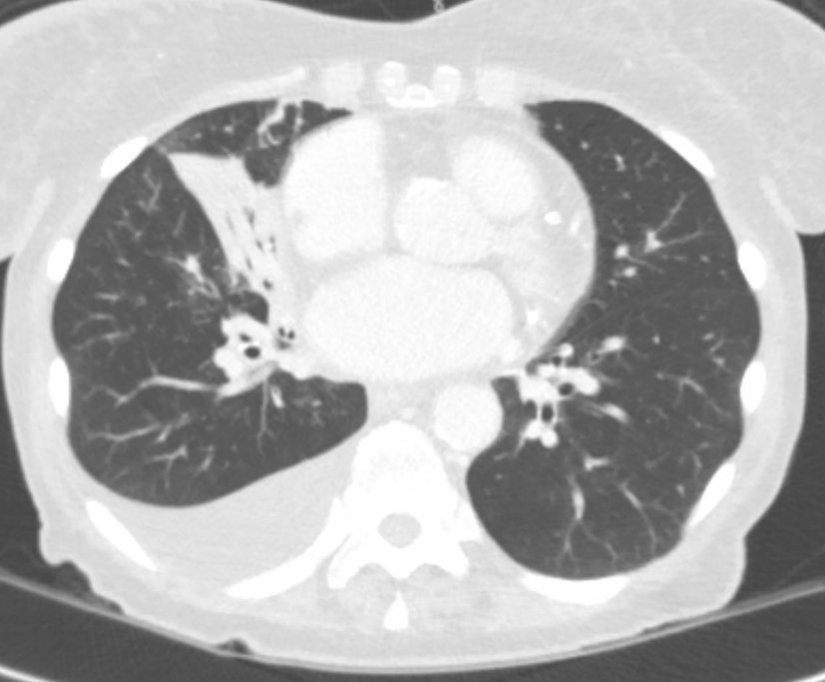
Axial CT image shows consolidation with air bronchogram in the right middle lobe and a right pleural effusion. Although the presence of these findings, the scan was categorized as CO-RADS 0 because it did not include the entire chest and therefore considered insufficient for assigning a score
